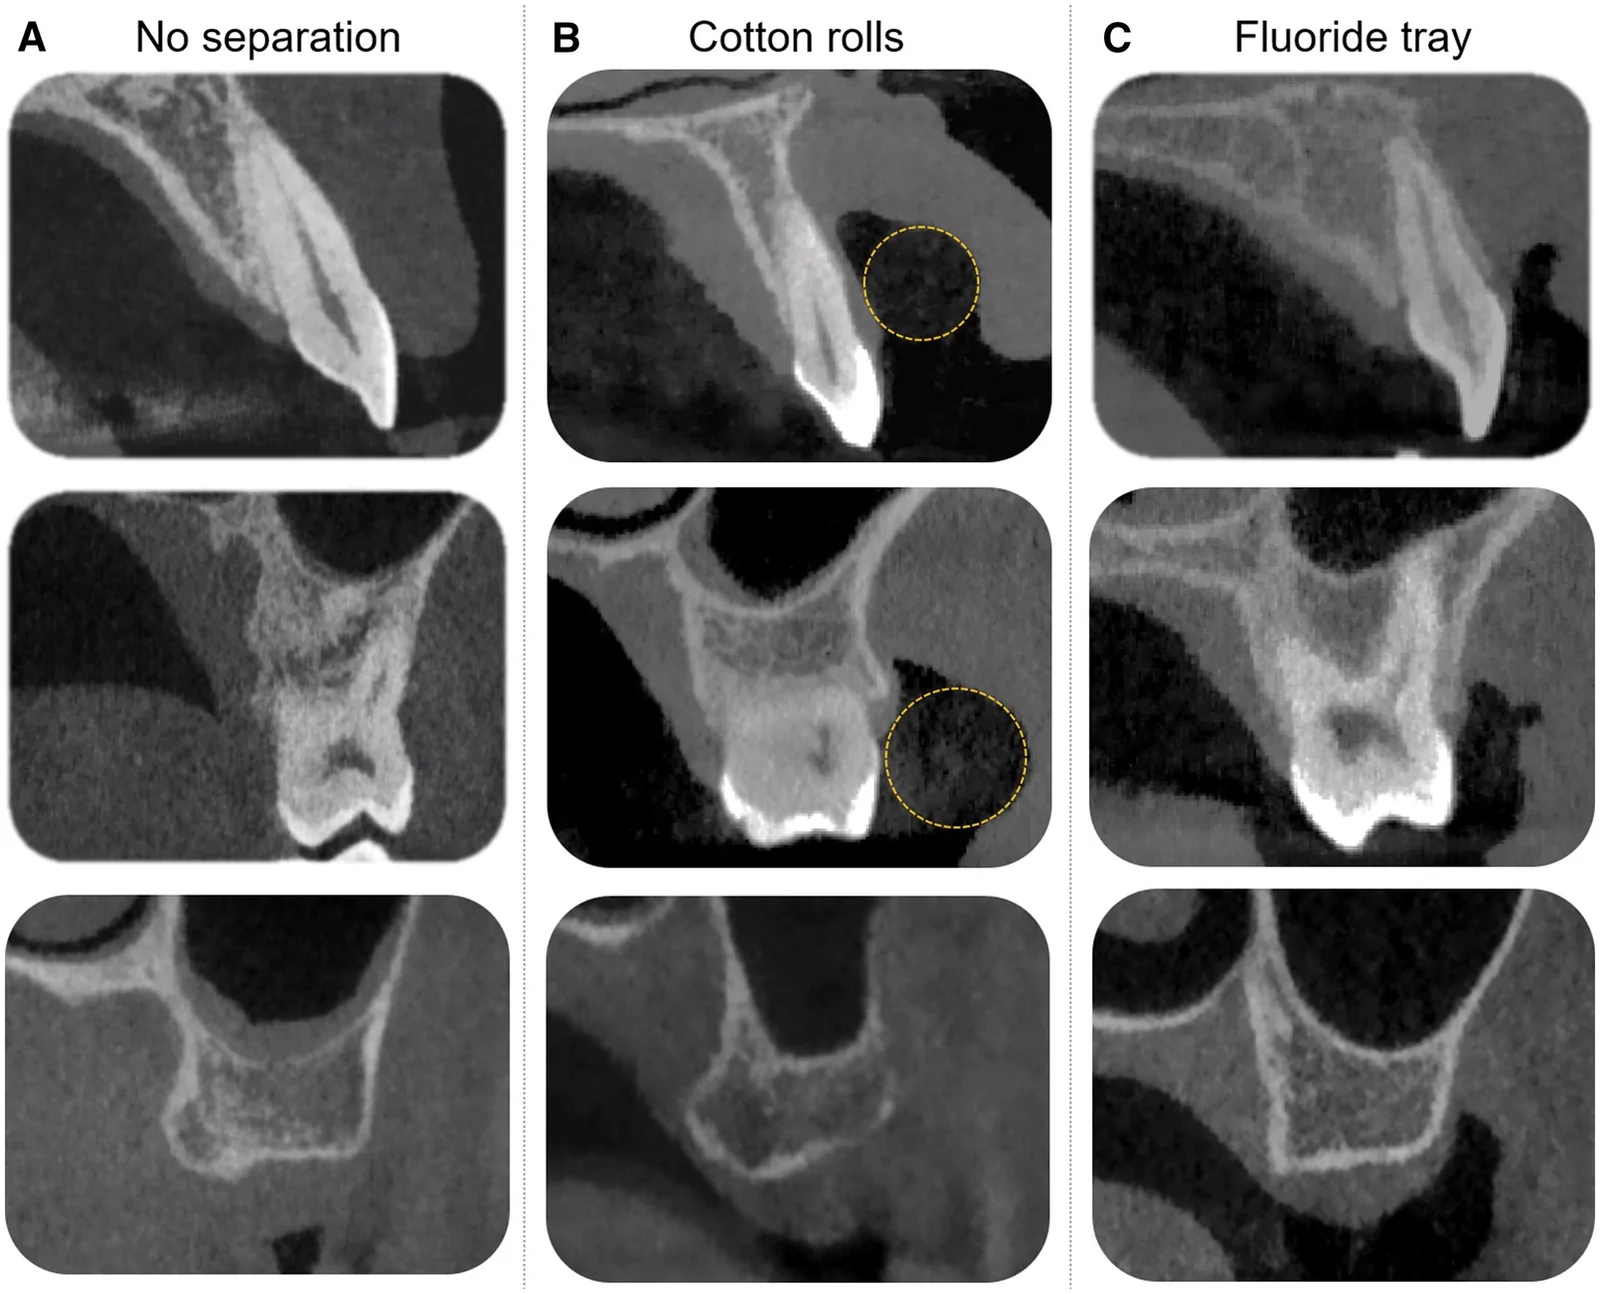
CBCT scans of the maxillary anterior region, posterior regions, and tuberosity obtained without soft tissue separation (A), with cotton rolls (B), and with a disposable fluoride tray (C). Yellow circles indicate the relative radiodensity of the cotton rolls.
